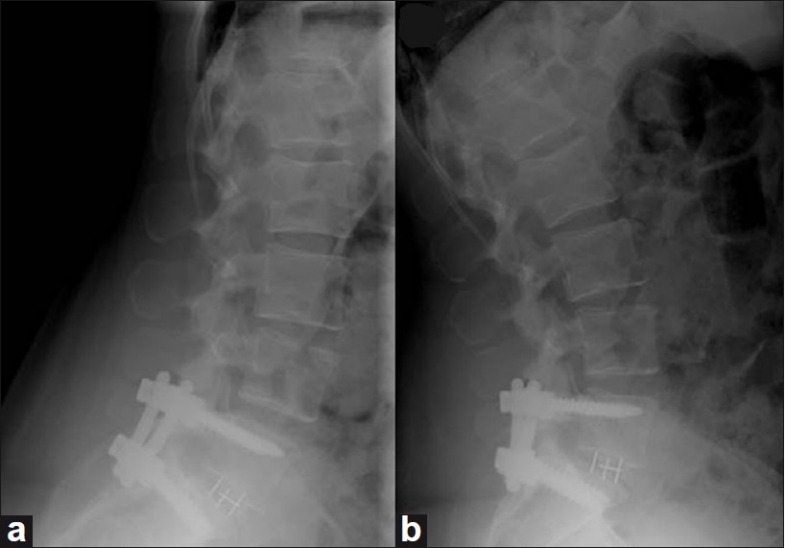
Lateral flexion (a) and extension (b) radiographs of the lumbar spine demonstrating pedicle screw instrumentation and interbody graft at L5–S1 with no significant motion between flexion and extension at the instrumented level
